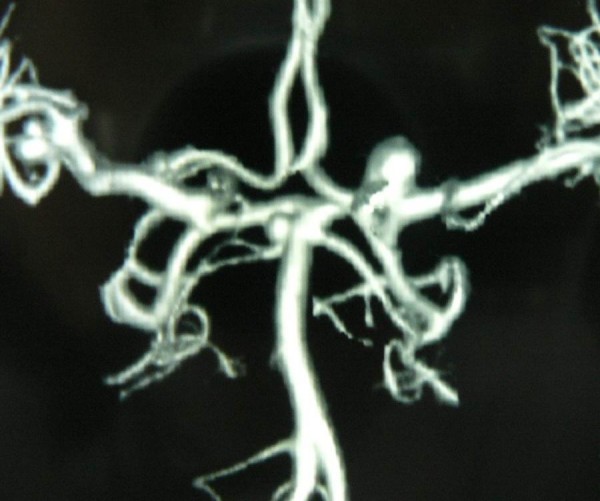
Three-dimensional reconstructed CT angiography of brain vasculature revealing multiple aneurysms: Right MCA, Left ICA bifurcation, and distal basilar artery.
